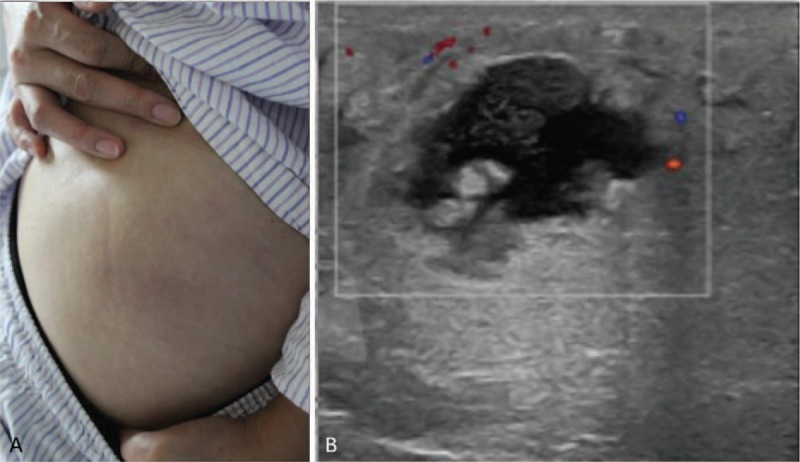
(A) Physical examination revealed a mass sized at 7.0 × 6.0 cm on her left buttock, accompanied by local red, swelling, and tenderness. (B) Ultrasonic examination of the mass disclosed the echo of the soft tissue layer is diffuse and the boundary changed to be unclear, and anechoic region was seen inside the mass.
